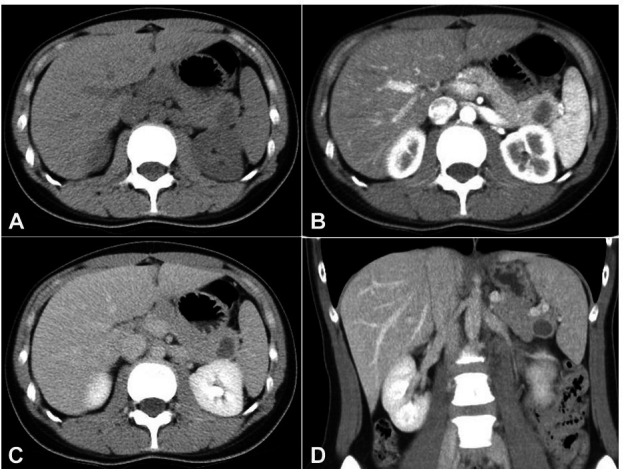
CT scan showed a hypodense and unilocular cyst in the tail of the pancreas, with no internal septation or calcification (A – plain, B – arterial phase, C – delayed phase, D – coronal section of delayed phase).
